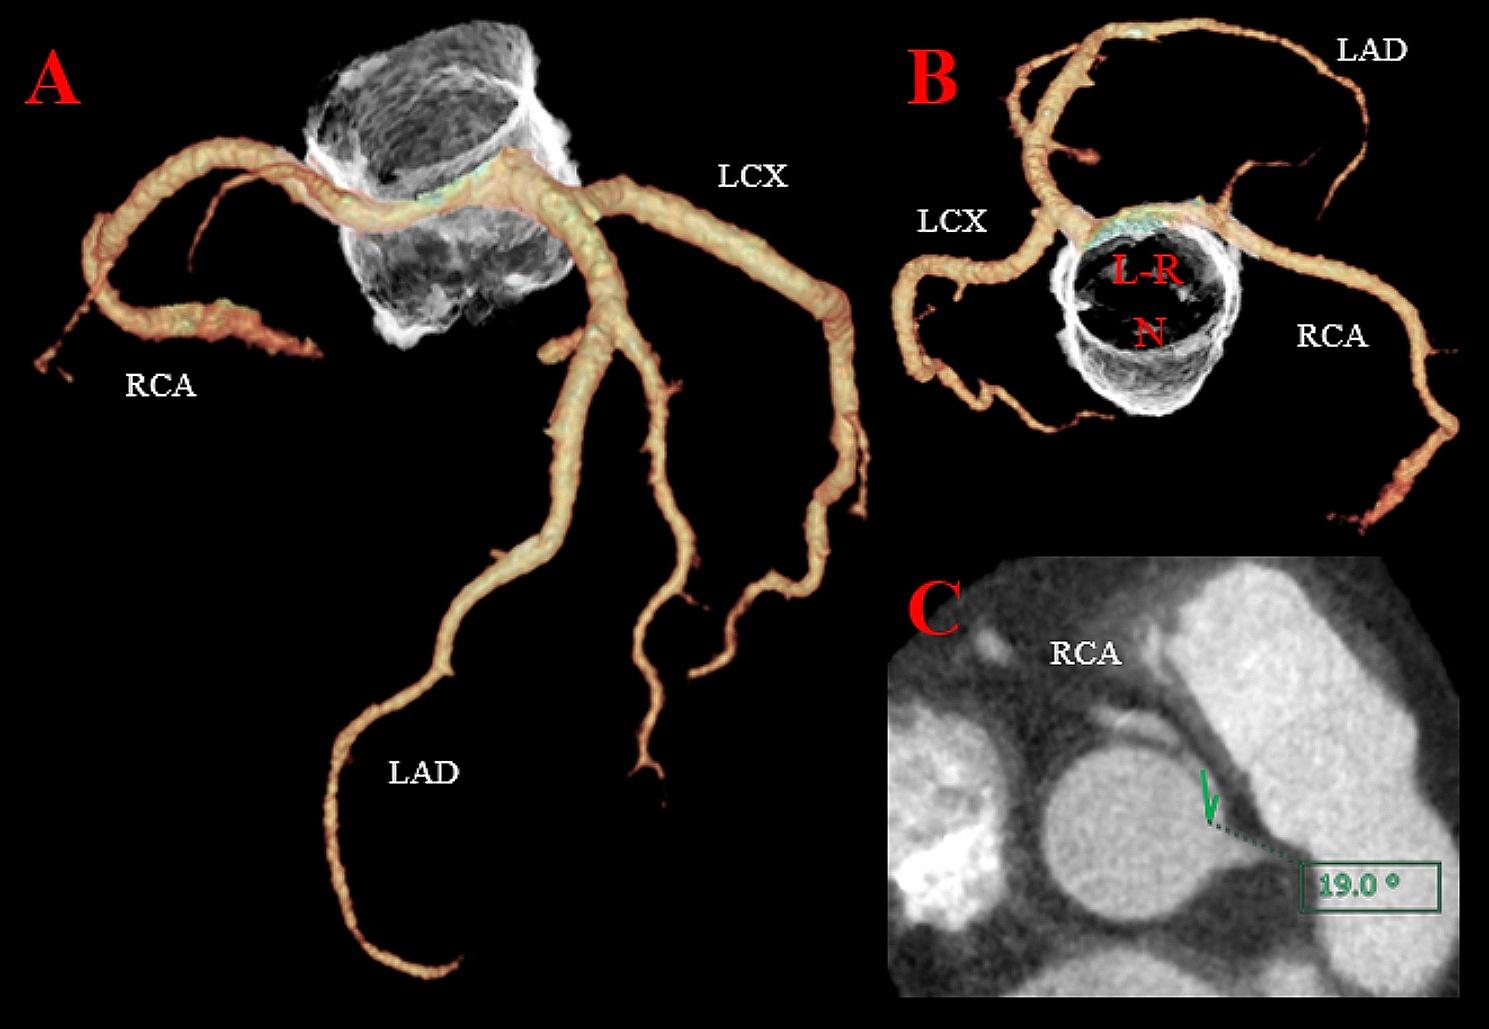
Preoperative cardiac computed tomography. (A) The right coronary artery originated from the left coronary ostium. (B) Surgeon’s view of the aortic valve. L-R: the fusion of left and right coronary cusps, N: noncoronary cusp (C) a take-off angle of the right coronary artery was 19°
